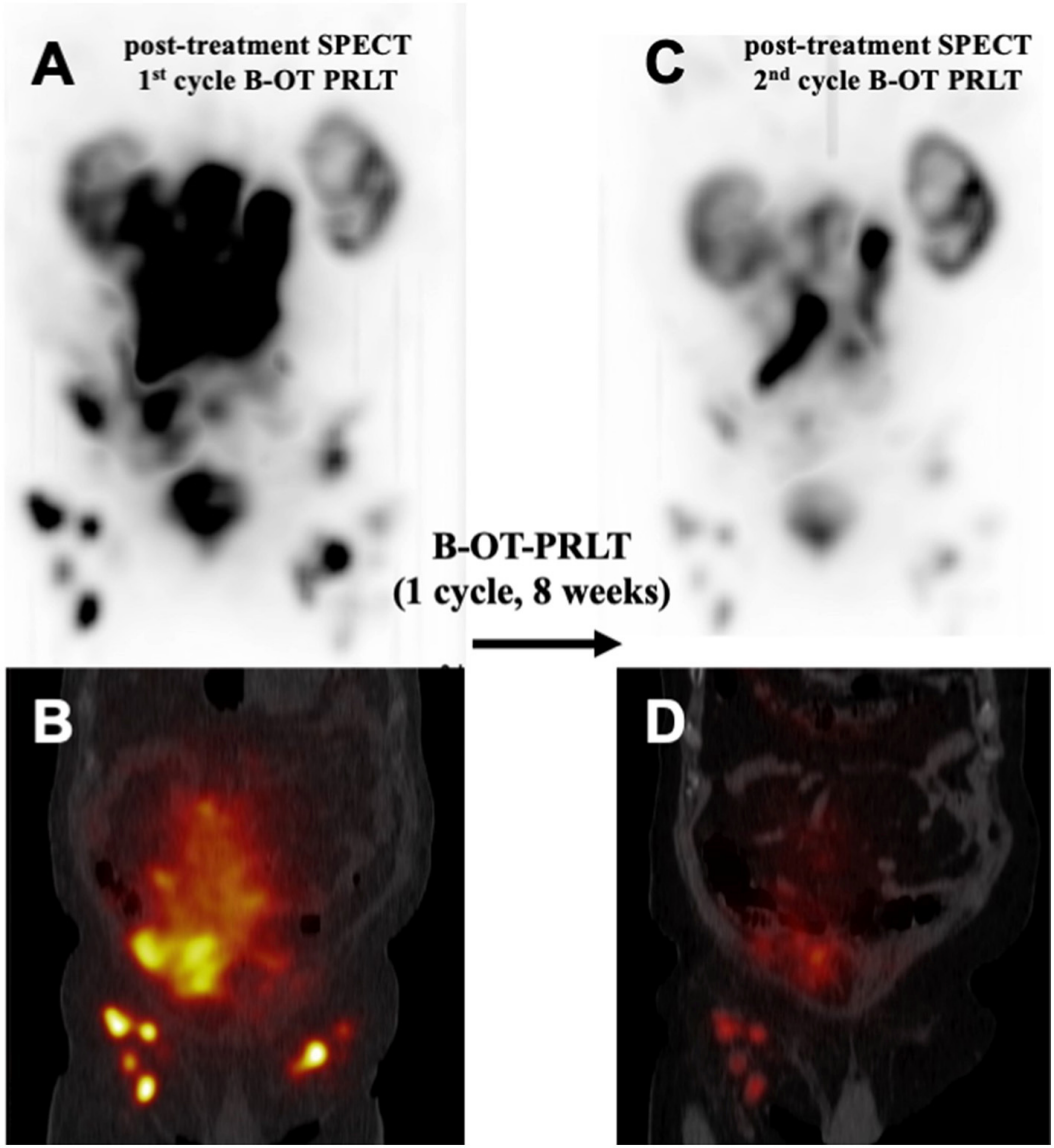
Comparison of post-treatment ^177^Lu-PSMA SPECT/CT examinations after one cycle of B-OT-PRLT: Post-treatment ^177^Lu-PSMA SPECT (A) and fused SPECT/CT image (B), representing the 1st cycle of B-OT-PRLT in 6/2020. After 8 weeks, the post-treatment ^177^Lu-PSMA SPECT (C) and the fused SPECT/CT image (D) of the 2nd cycle of B-OT-PRLT in 7/2020 showed clearly partial remission of the disease.
